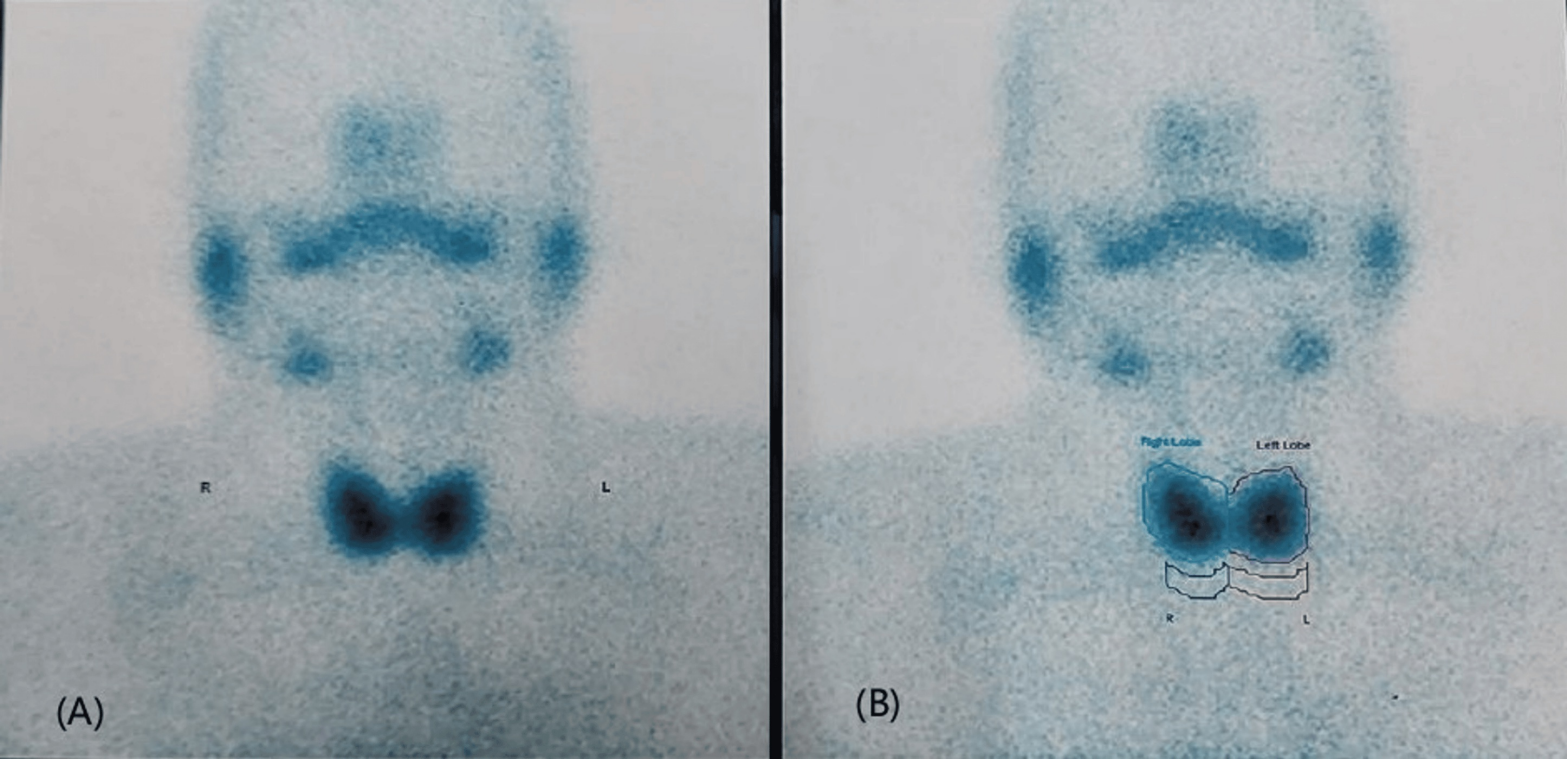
Thyroid scintigraphy with technetium-99m pertechnetate. (A) Anterior planar image showing diffuse, homogeneous tracer uptake in both lobes of the thyroid gland. (B) Same image with regions of interest drawn around each lobe for uptake quantification, showing a calculated thyroid uptake of 1.2%.
